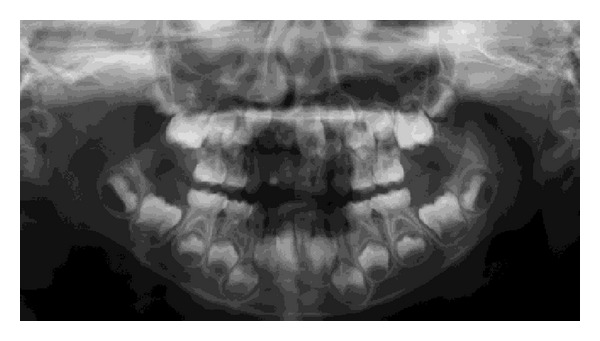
Panoramic radiograph at first visit (case 2). The permanent maxillary right central and lateral incisors are malformed and hypocalcified.
